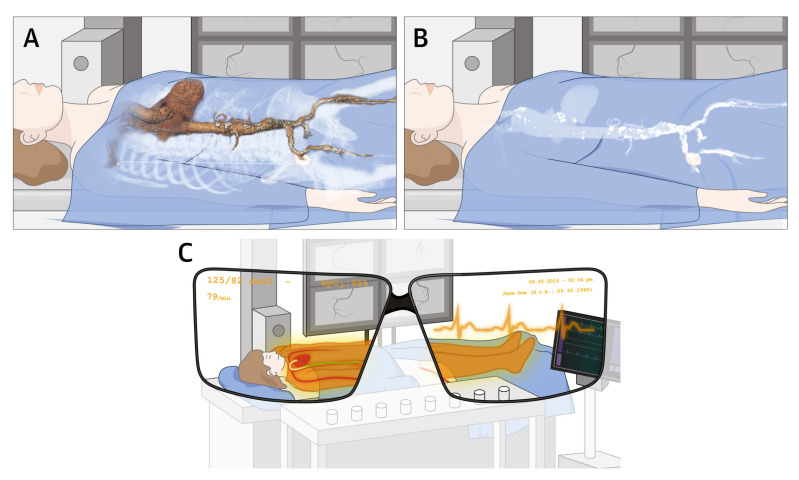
AR integrates superimposed virtual elements into a real-world environment. (A) 3D CT image of a patient’s vasculature could be imaged by an operator or (B) vascular calcifications could be focused to guide the best puncture site and avoid complications during the procedure. (C) AR superimposes virtual elements into a real-world environment. Reprinted with permission from Jun et al. [173].
